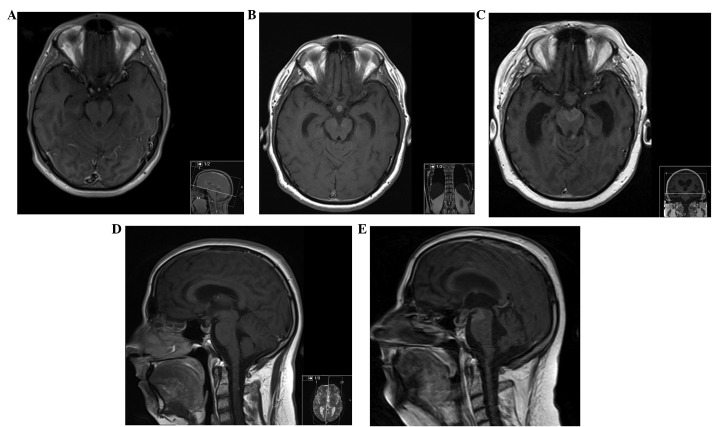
MRI of the brain. (A) Axial section, contrast-enhanced T1-weighted MRI of the brain, at presentation, demonstrating no lesions. (B and D) Axial and sagittal section, respectively, contrast-enhanced T1-weighted MRI of the brain, at 6 months after initial presentation, showing a metastatic deposit in the pituitary stalk. (C) Axial and (E) sagittal section contrast-enhanced T1-weighted MRI of the brain, at 17 months after initial presentation, demonstrating new metastatic deposits in the interpeduncular cistern and in the left superior cerebellar peduncle. MRI, magnetic resonance imaging.
